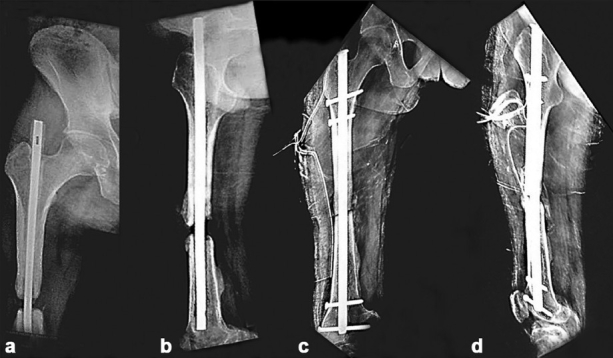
Anteroposterior (a) and lateral (b) radiograph of right thigh including hip depicting a gap non-union of femur with infection treated with antibiotic impregnated intramedullary nail. Anteroposterior (c) and lateral (d) radiograph of the same patient showing exchange interlock nailing performed after control of infection
